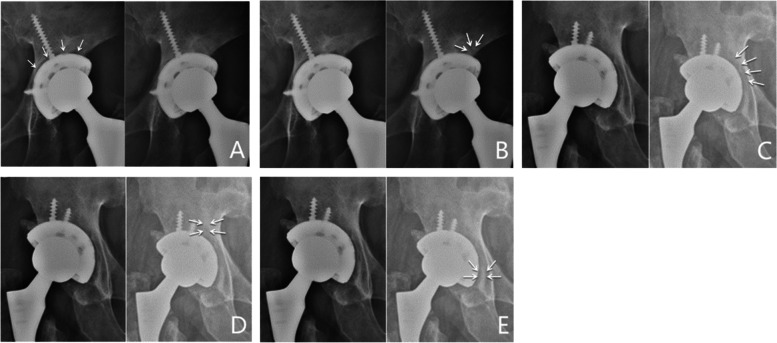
Representative cases with radiographic signs of osseointegration as defined by the Anderson Orthopedic Research Institute, inlcluding absence of RLL A presence of superolateral buttresses B presence of medial stress-shielding C presence of radial trabeculae D and presence of inferomedial buttresses E. RLL: radiolucent line
